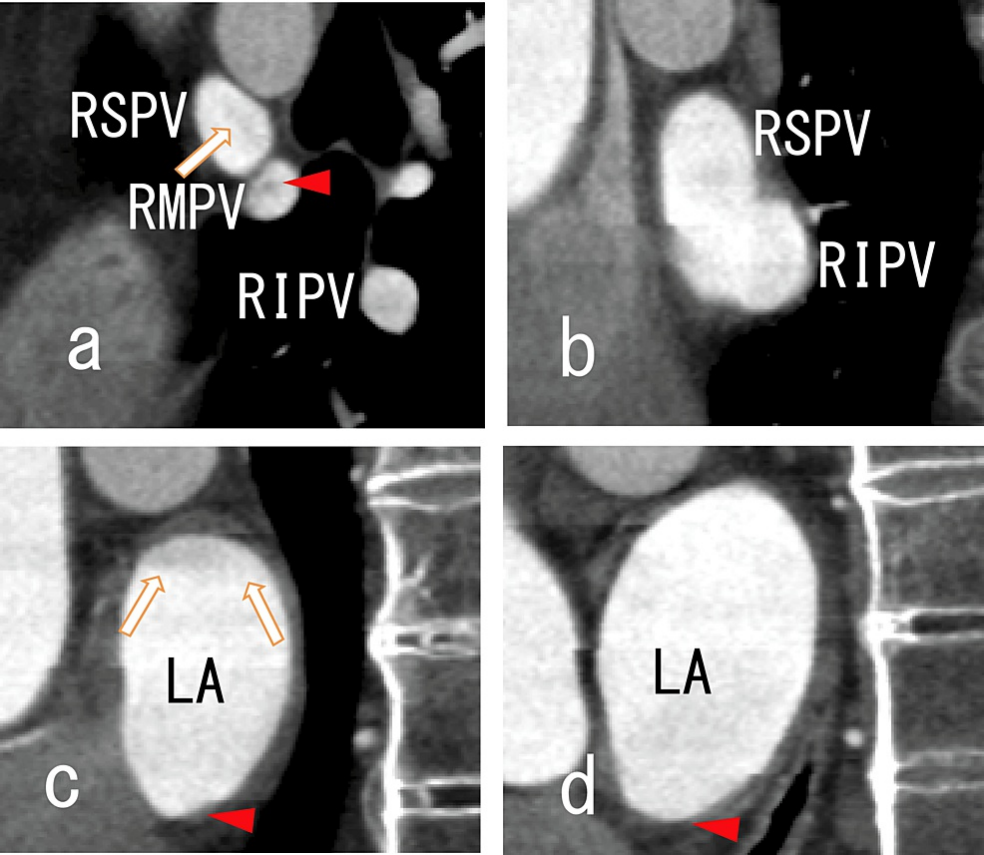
Sagittal images from an 80-MDCT scan obtained after five months of standard-dose warfarin remedy Sagittal images from an 80-MDCT scan obtained after five months of standard-dose warfarin remedy showing thrombi in the RSPV, RMPV, RIPV and LA. (a) There were rather dark areas in the RSPV (arrow), suggesting that the thrombi were partly resolved. There were rather dark areas in the RMPV (arrowhead), suggesting that there were thrombi in the RMPV. There were dim dark areas in the RIPV dimly. All the dark areas in the vessels became slightly clearer, suggesting that all the thrombi had partly resolved. (b) After the RSPV’s connection to the RMPV, the RSPV then linked to the RIPV. There were rather dim, dark areas in the RSPV and RIPV, suggesting that the thrombi had partially resolved. (c) There were thin, vague, dark areas in the superior region of the LA (arrows), suggesting that the thrombi in the LA had partially resolved. There were no new dark areas in the inferior region of the LA (arrowhead). (d) There were no dark areas in the LA. There were no new dark areas in the inferior region of the LA (arrowhead). LA, left atrium; RIPV, right inferior pulmonary vein; RMPV, right middle pulmonary vein; RSPV, right superior pulmonary vein; 80-MDCT, 80-slice multidetector computed tomography
